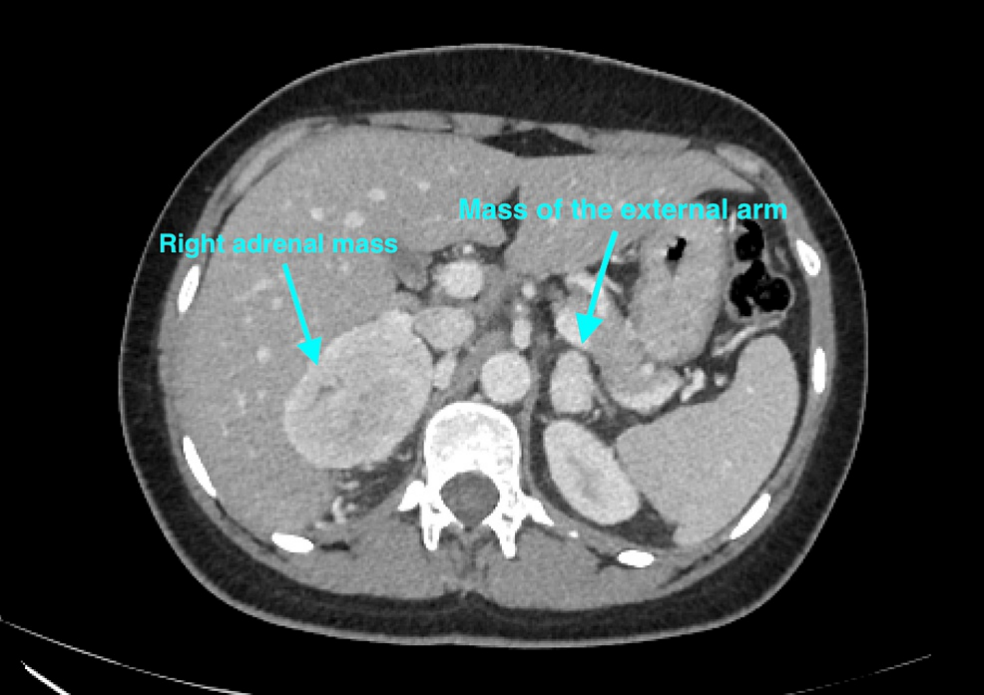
Cross section of an abdominal CT scan showing one right adrenal mass 55 × 70 × 50 mm in diameter and the other at the level of the medial arm of the left adrenal measuring 21 × 15 × 19 mm CT: computed tomography
